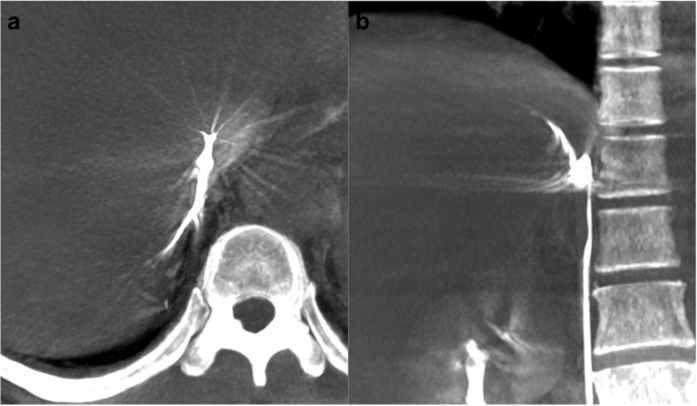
A 48-year-old woman with primary aldosteronism and a right adrenal nodule. The right inferior phrenic vein was shown on Dyna computed tomography maximum intensity projection imaging in the transverse plane (a) and coronal plane (b).
